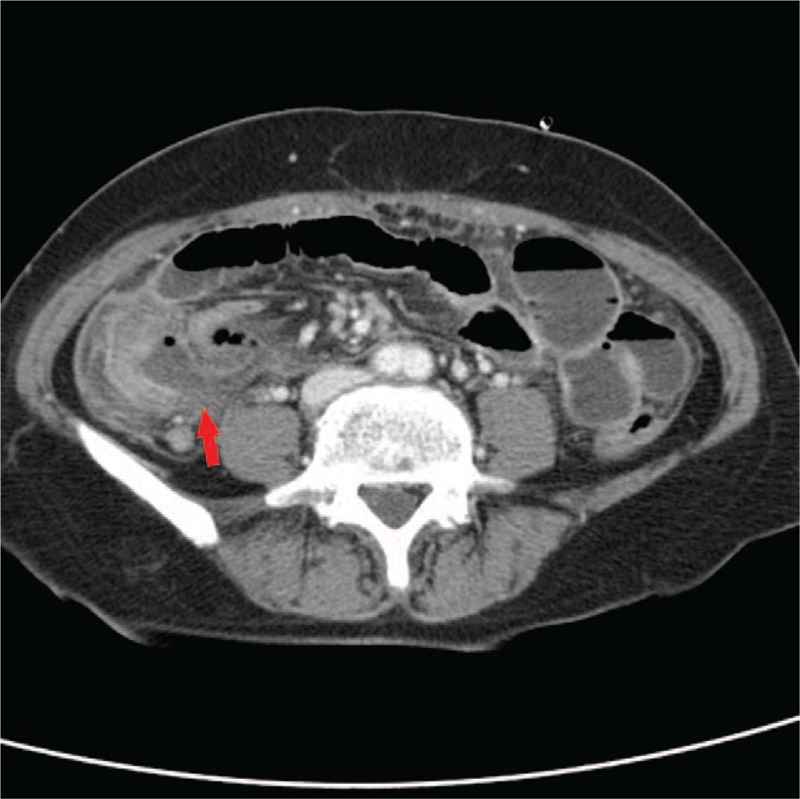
Contrast-enhanced abdominal and pelvic computed tomography in case 5. A pericecal inflammatory infiltration with abscess and bowel edema (arrow).
